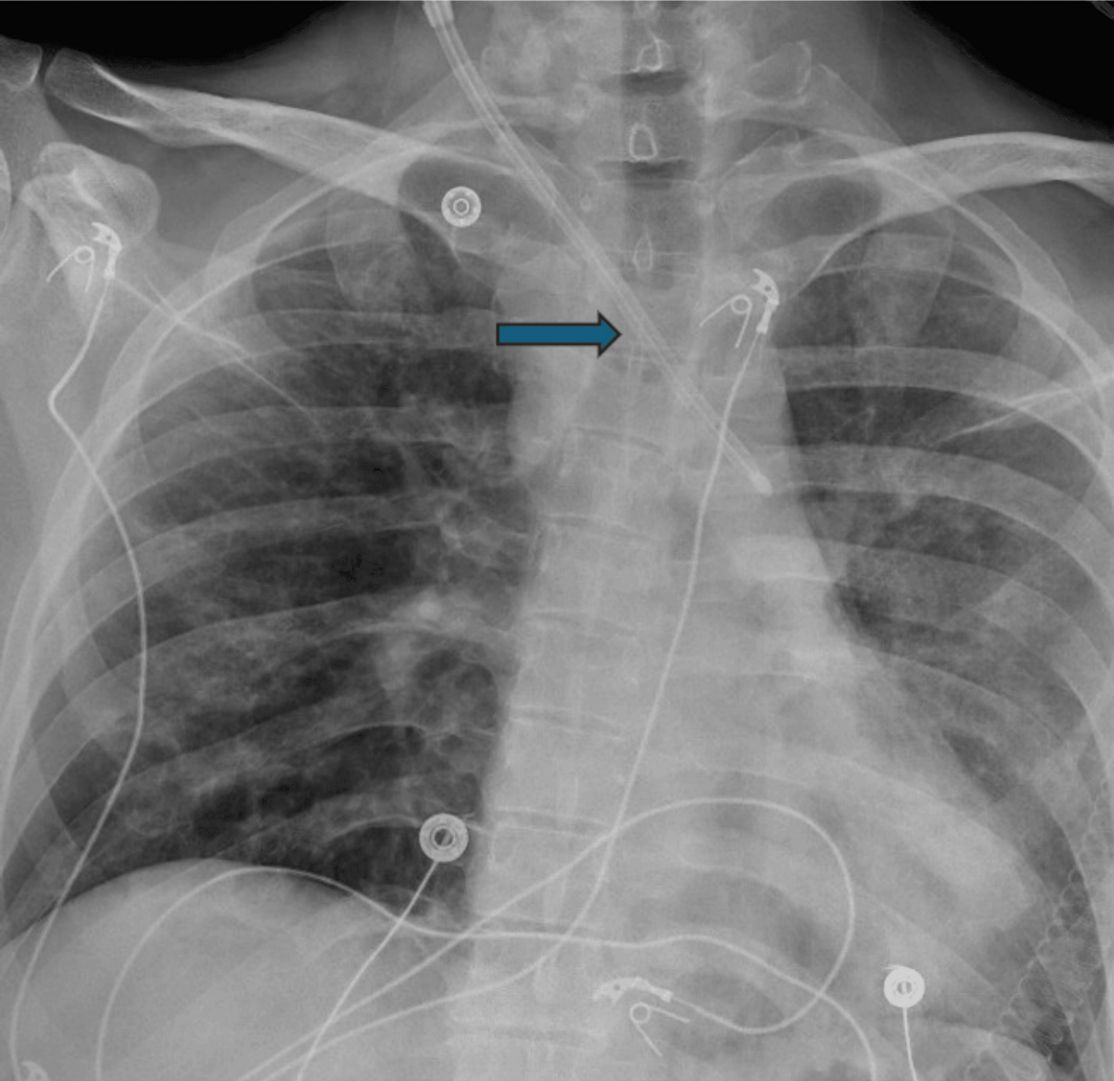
A chest AP radiograph showed a central venous catheter placement in the subclavian artery with its tip ending in the aortic arch (arrow). AP: anterior posterior
